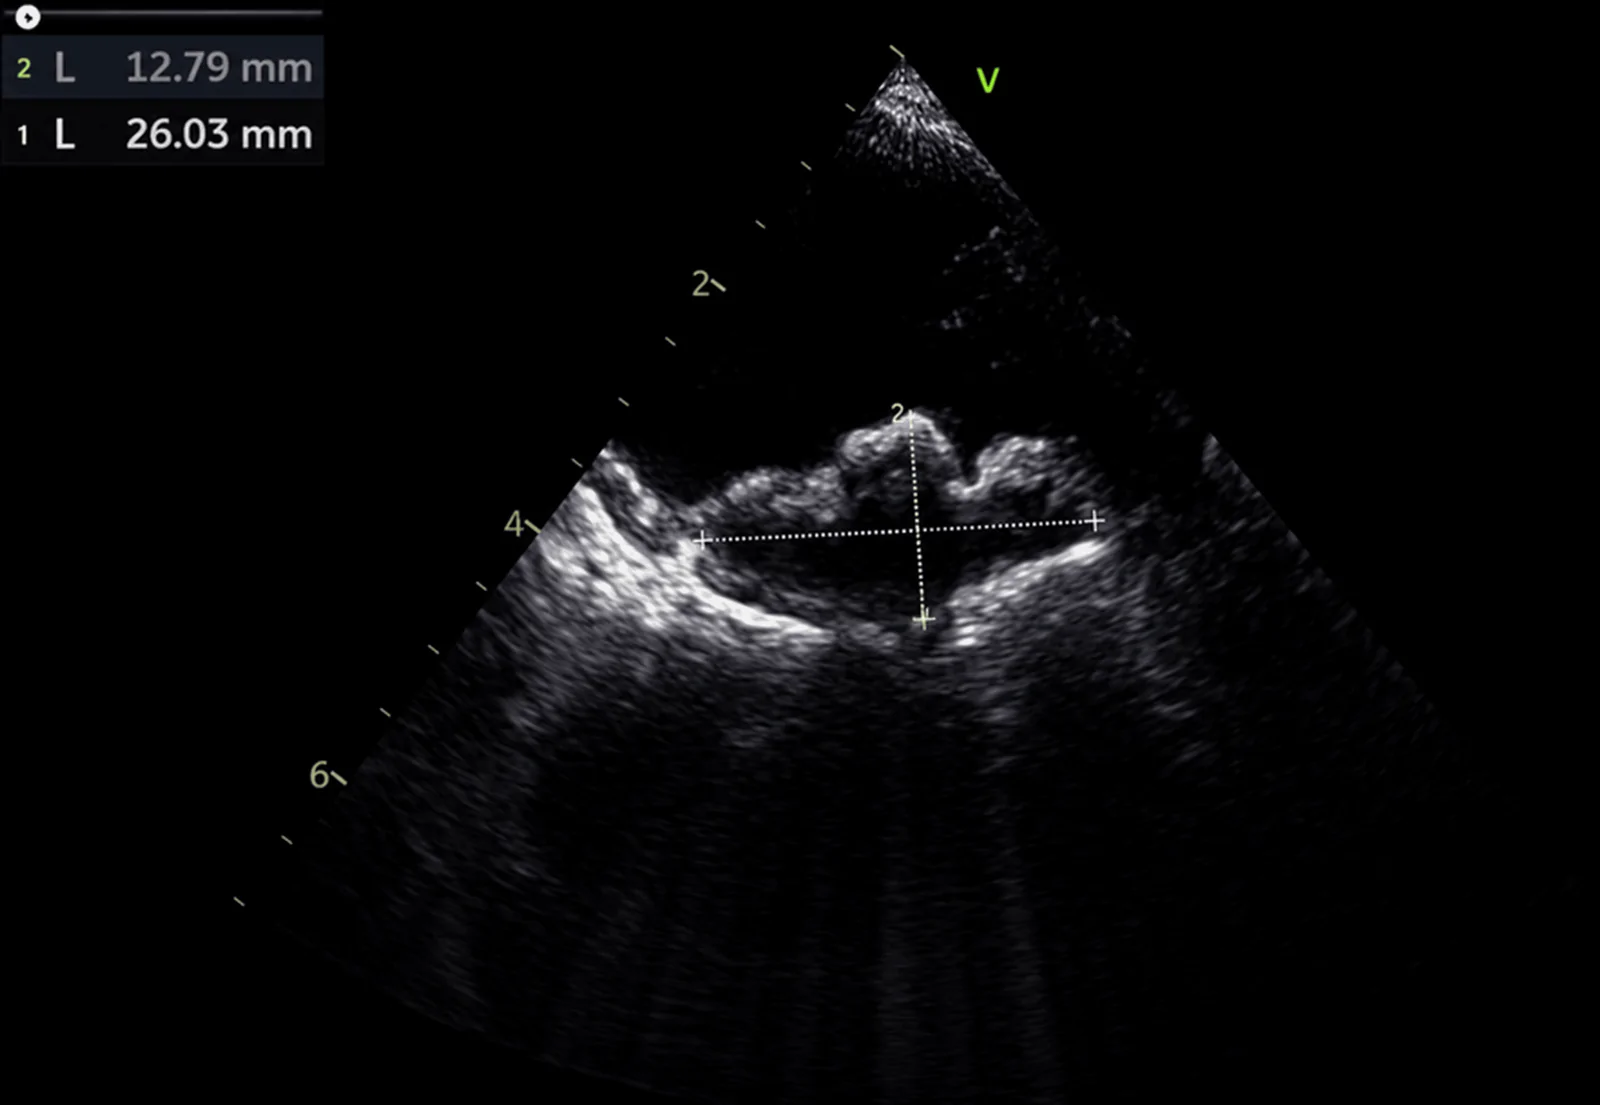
Transesophageal echocardiographic transverse view of the distal aortic arch showing a large, markedly irregular, protruding atheromatous plaque measuring 26.03 × 12.79 mm
